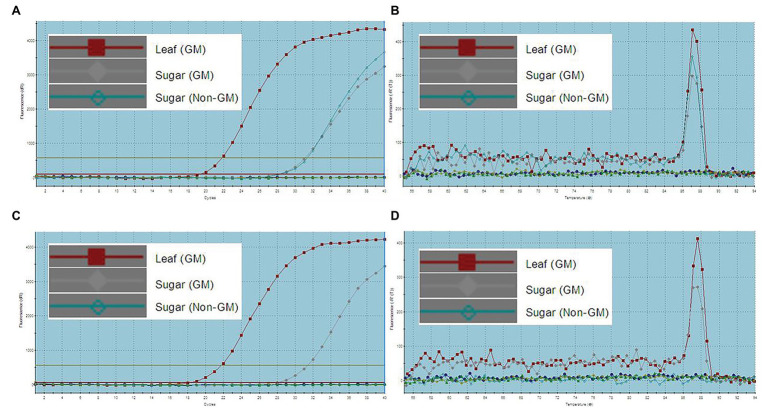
RT-qPCR analysis of GM and non-GM sugar. (A): amplification plots of endogenous gene *actin* (CT value: GM leaf, 22.12; GM sugar, 31.42; non-GM sugar, 31.17); (B): dissociation curve of endogenous gene *actin*, the three samples share the same dissociation curve; (C): amplification plots of the endogenous gene *Cry1Ab* (CT value: GM leaf, 21.81; GM sugar, 31.44; non-GM sugar, no CT value); (D): dissociation curve of the endogenous gene Cry1Ab, GM leaf sample and GM sugar share the same dissociation curve, but the non-GM sugar has no dissociation curve.
